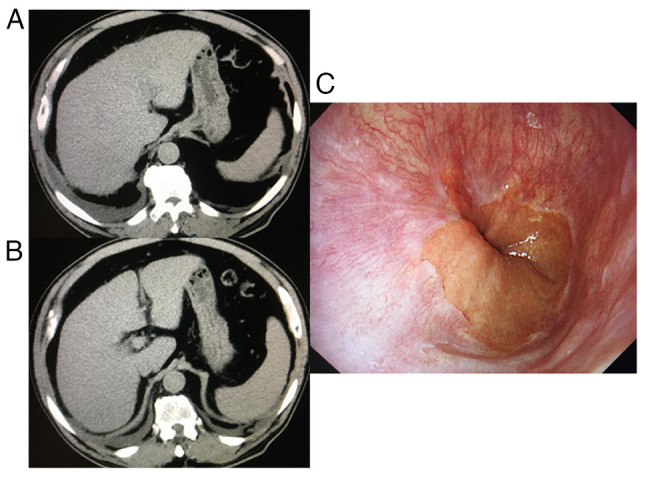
Abdominal CT scan and upper gastrointestinal endoscopic images. (A and B) Abdominal CT scan indicating signs of liver cirrhosis with paraumbilical vein dilatation, mild splenomegaly and right pleural effusion. (C) An upper gastrointestinal endoscopic examination did not reveal any esophageal varices. CT, computed tomography.
